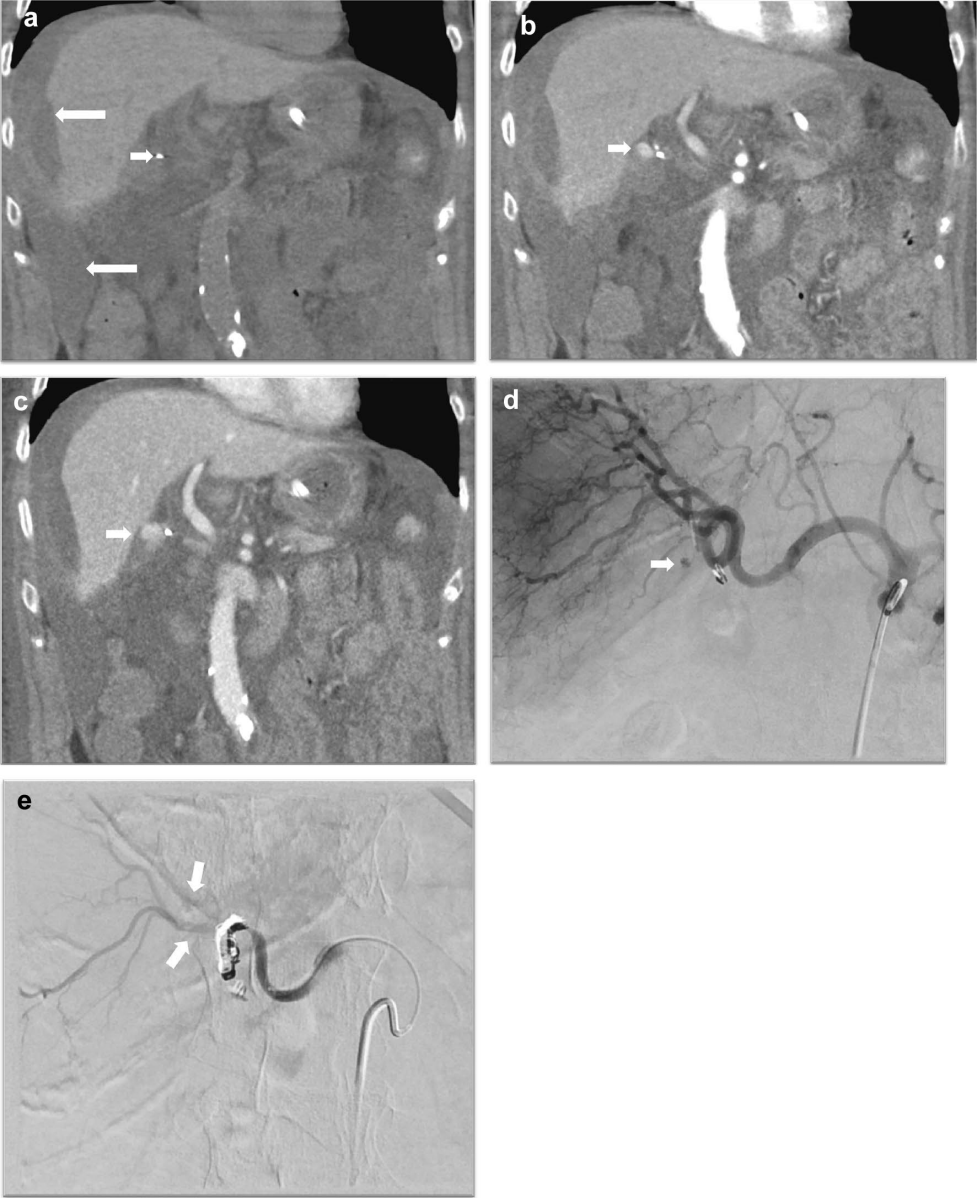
A 70-year-old male status post cholecystectomy, with bleeding from cystic artery stump. CT Abdomen was obtained 10 days post-cholecystectomy for down-trending hemoglobin. a, b and c Coronal non-contrast, arterial, and venous phase images, respectively, showing active expanding contrast extravasation (short arrows in images b and c) adjacent to a clip at the surgical site (short arrow in image a). Associated large hemoperitoneum (long arrows in image a). d The catheter celiac angiogram demonstrated a contrast blush adjacent to the clip, which was arising from the proximal right hepatic artery (arrow) in the region of the cystic artery stump. e Status post coil embolization of the proximal right hepatic artery with collateral flow opacifying the distal branches (arrows)
